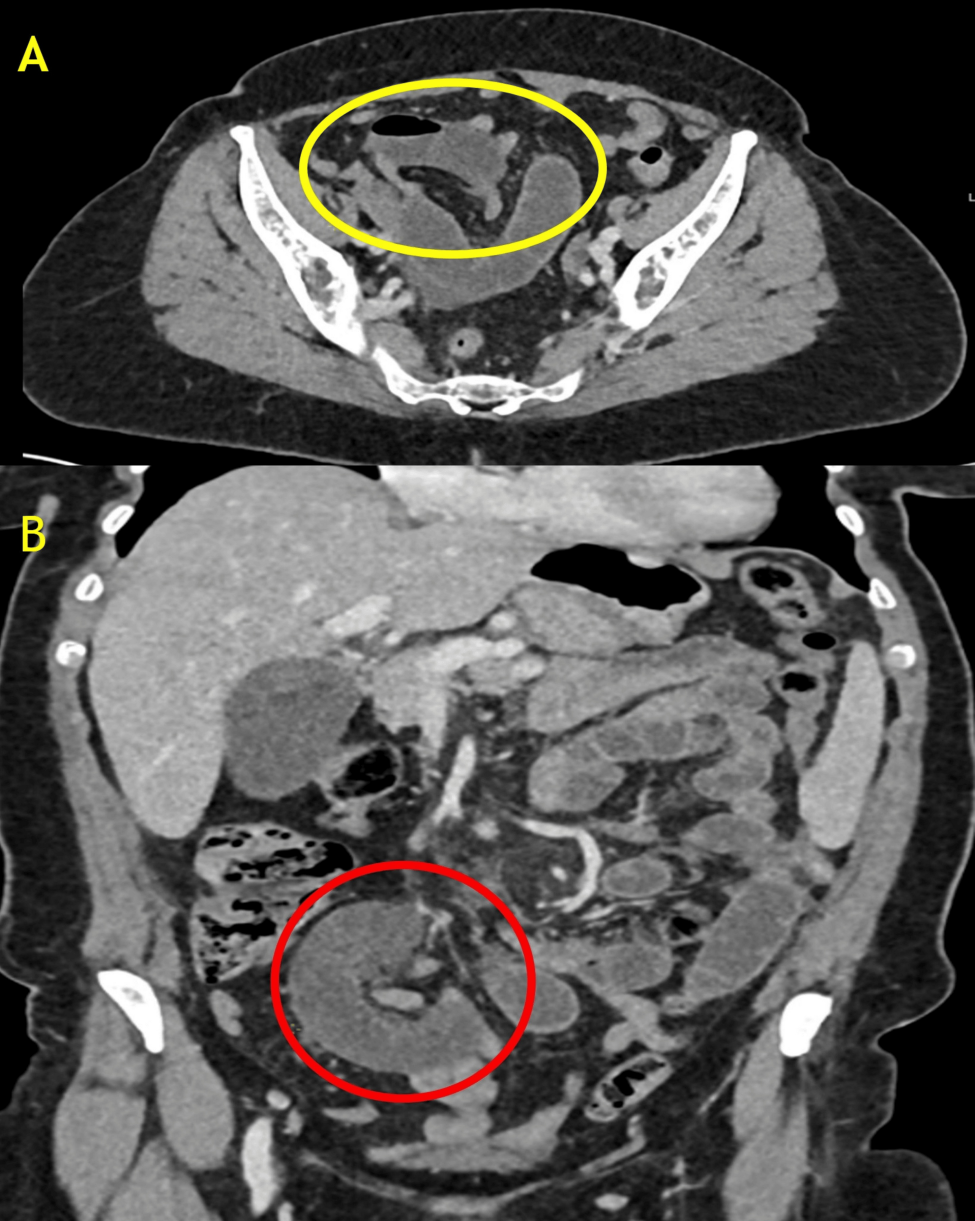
Contrast-enhanced CT images demonstrating small bowel closed-loop obstruction. (A) Axial section showing clustered, dilated small bowel loops with a characteristic "U-shaped" configuration, suggestive of a closed-loop obstruction (yellow circle). (B) Coronal section highlighting the transition point with mesenteric congestion and a radial distribution of mesenteric vessels converging towards the site of obstruction (red circle), consistent with internal herniation.
